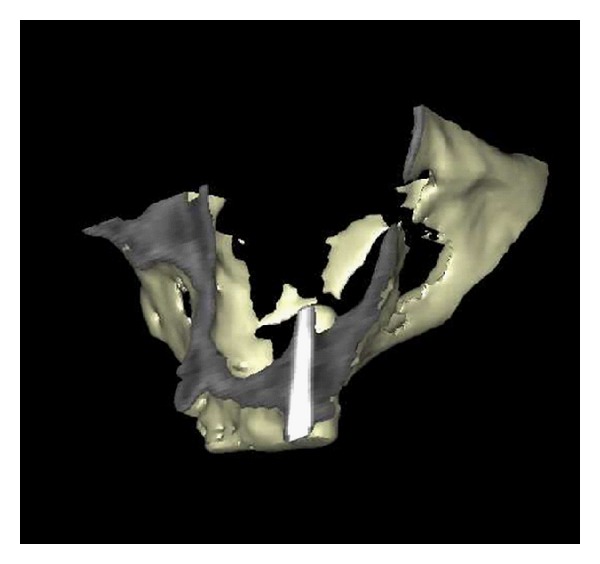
3 D reconstruction of CT scan from the same patient as in Figure 1 six months postoperatively, left side. Bone is formed around implants in the maxillary sinus floor.
